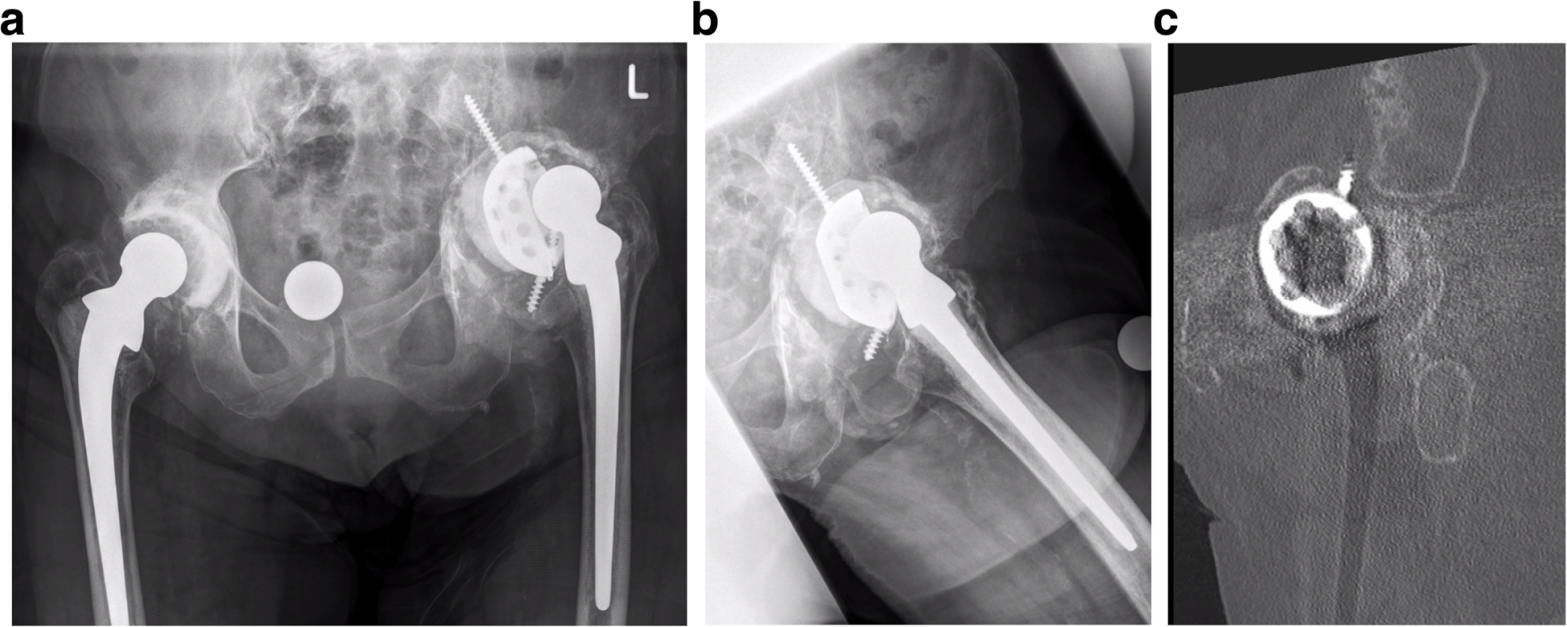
a X-ray of an 86-year-old woman who underwent first THA with a monobloc stem and a 33-mm head (Fa. Link) in 1973. In 1998, revision arthroplasty was performed with an oblong revision cup (LOR) for a high defect on the acetabular side with a well-fixed cemented stem. b Acetabular defect with a high rotation center. c CT data of the acetabular defect correlated with pelvic discontinuity
